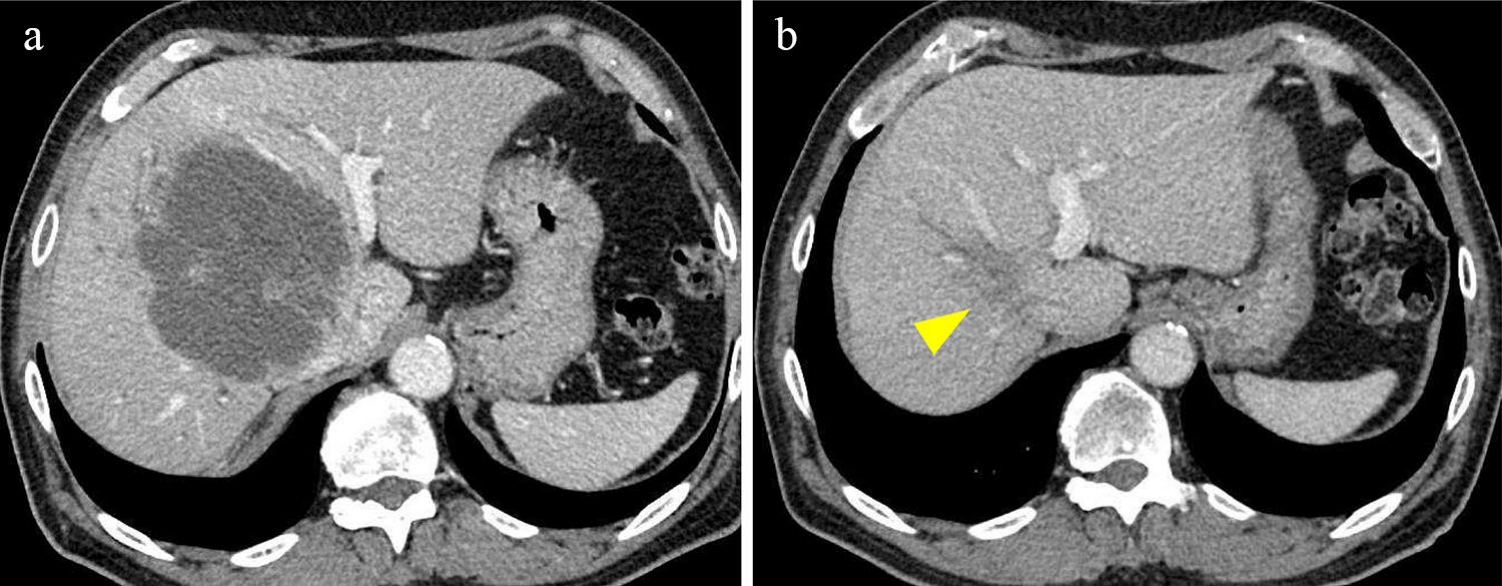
Contrast-enhanced computed tomography image. a Image of progressive disease after GCS therapy. Portal vein umbilicus slightly shifted to the left due to an enlarged tumor lesion. b After three 40 cycles of pembrolizumab, the primary tumor (yellow arrow) showed a remarkable reduction. GCS, gemcitabine (GEM) + concurrent cisplatin (CDDP) + adjuvant tegafur-gimeracil-oteracil (S-1)
